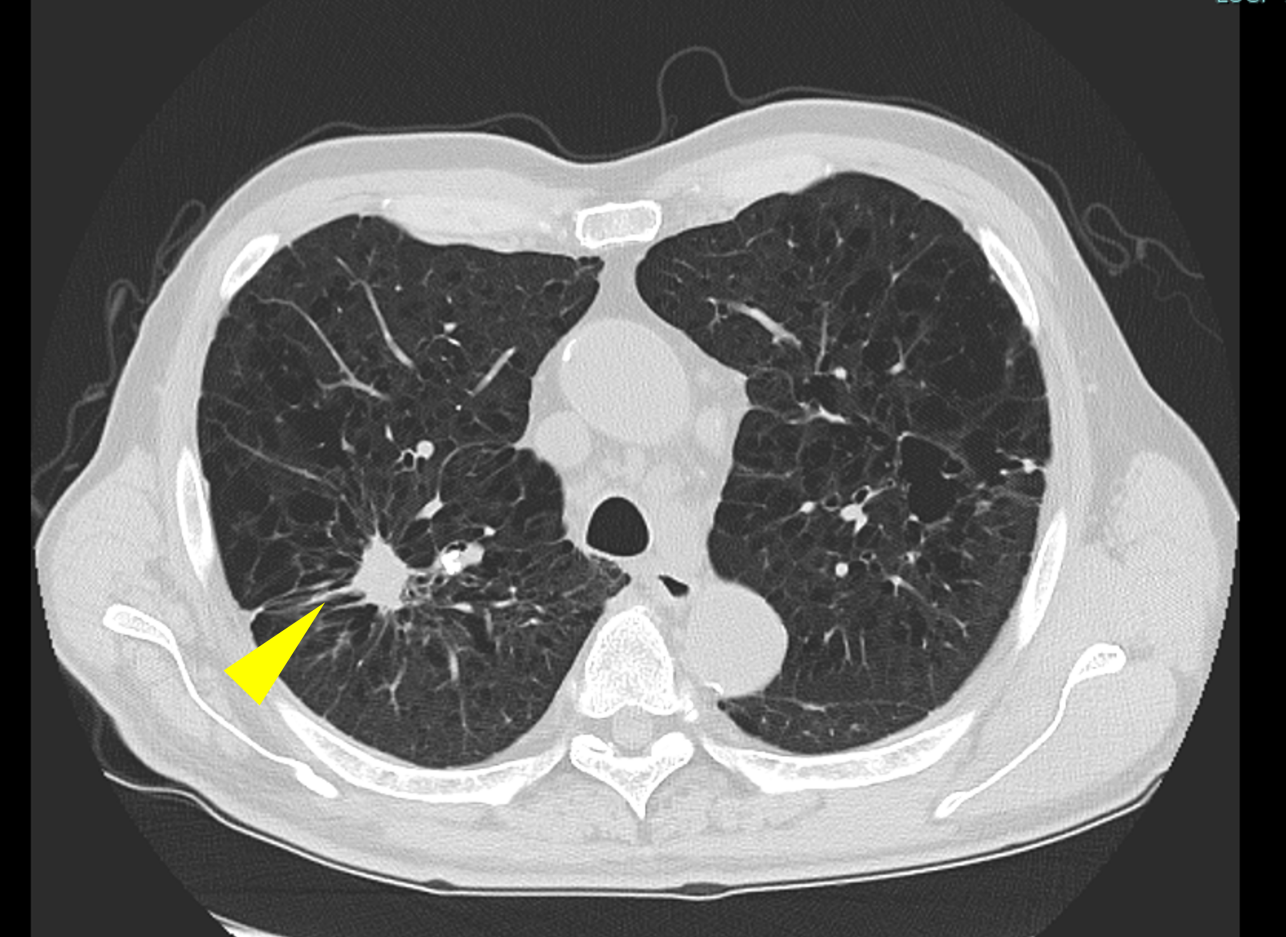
Chest computed tomography scan demonstrates a solitary silicotic nodule in the upper lobe of the right lung (yellow arrow).
